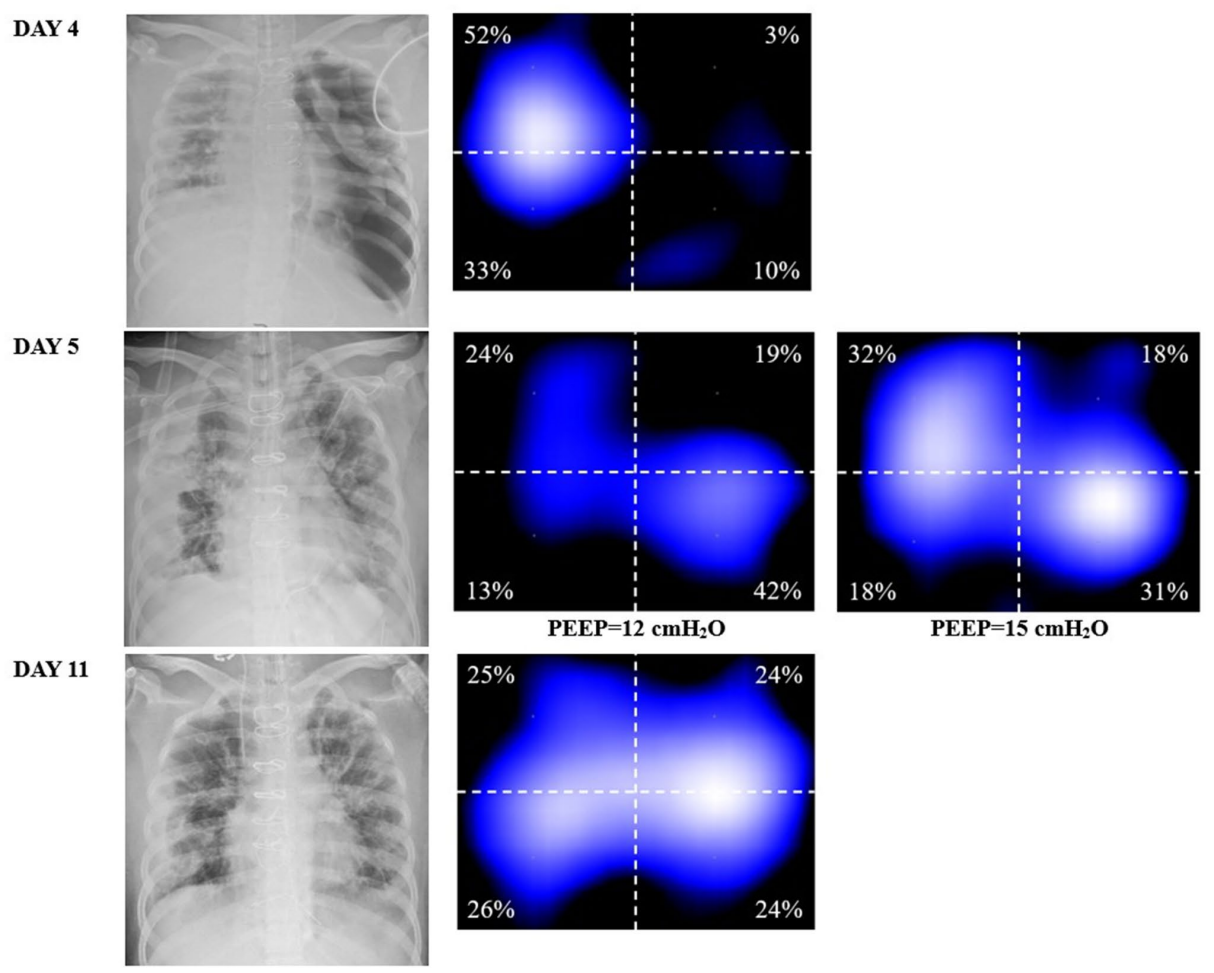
DAY 4, the chest X-ray indicating tension pneumothorax and the corresponding EIT ventilation image, showing a significant decrease in ventilation in the left lung. DAY 5, chest X-ray (at a PEEP of 6 cmH2O) shows left lung reopening after transthoracic drainage, but right lung atelectasis. At a PEEP of 15 cmH2O, the right lung was better ventilated compared to that at 12 cmH2O (well ventilated regions in light blue in EIT). DAY 11, improvement in exudation and atelectasis, the left side chest drain has been removed, and the homogeneity of ventilation in both lungs has been improved compared to the previous status.
